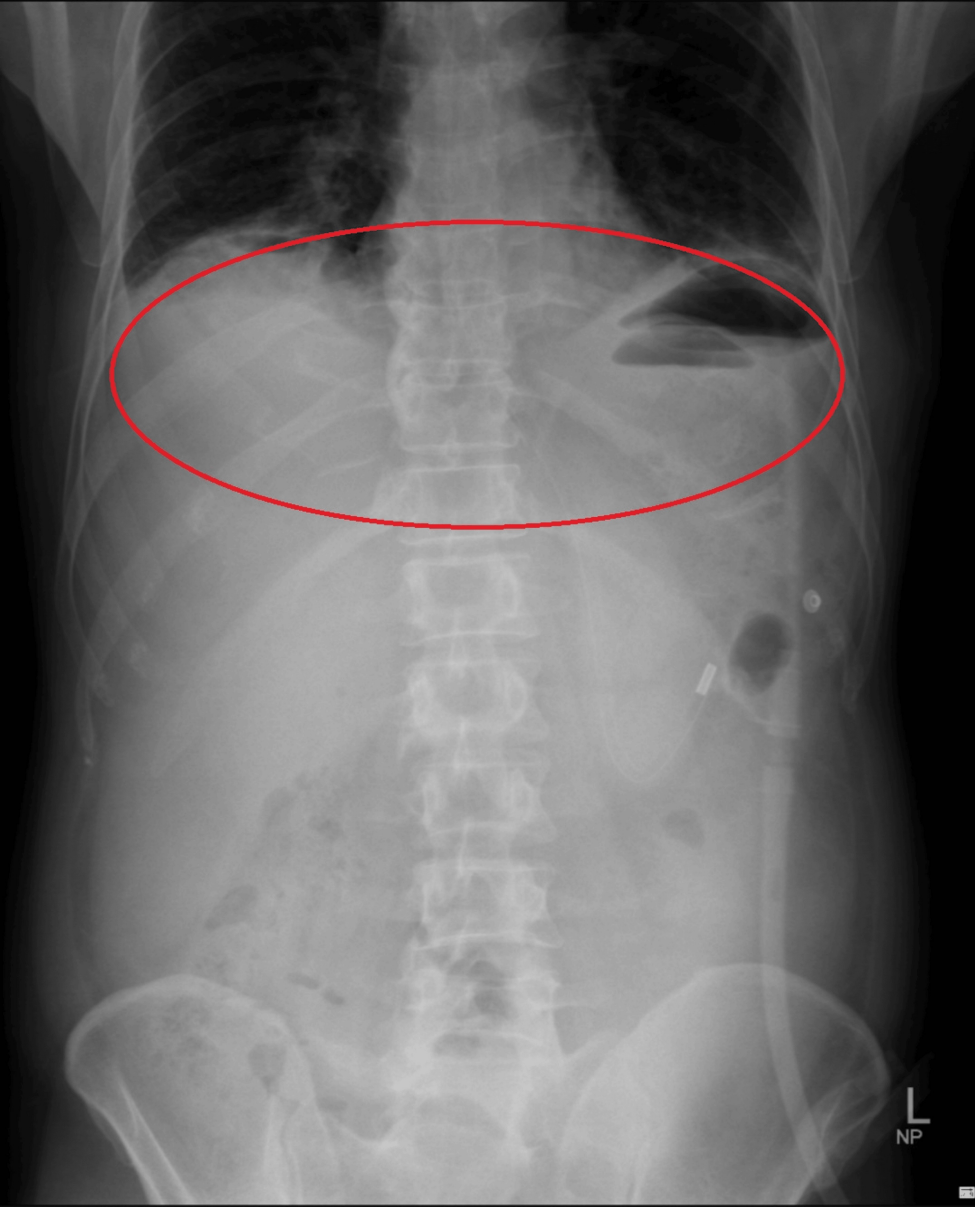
Abdomen X-ray showing air under diaphragm The marked area shows air under the diaphragm
